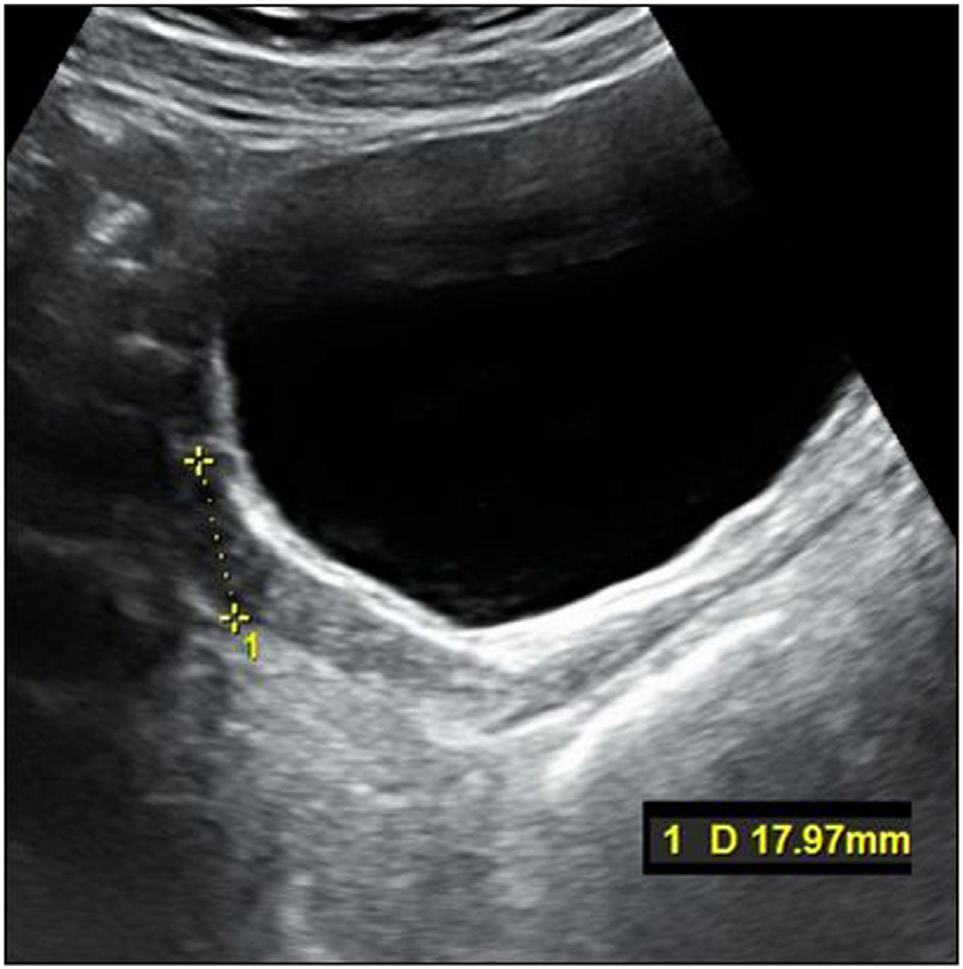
Ultrasonography of uterus at the initiation of the treatment.
